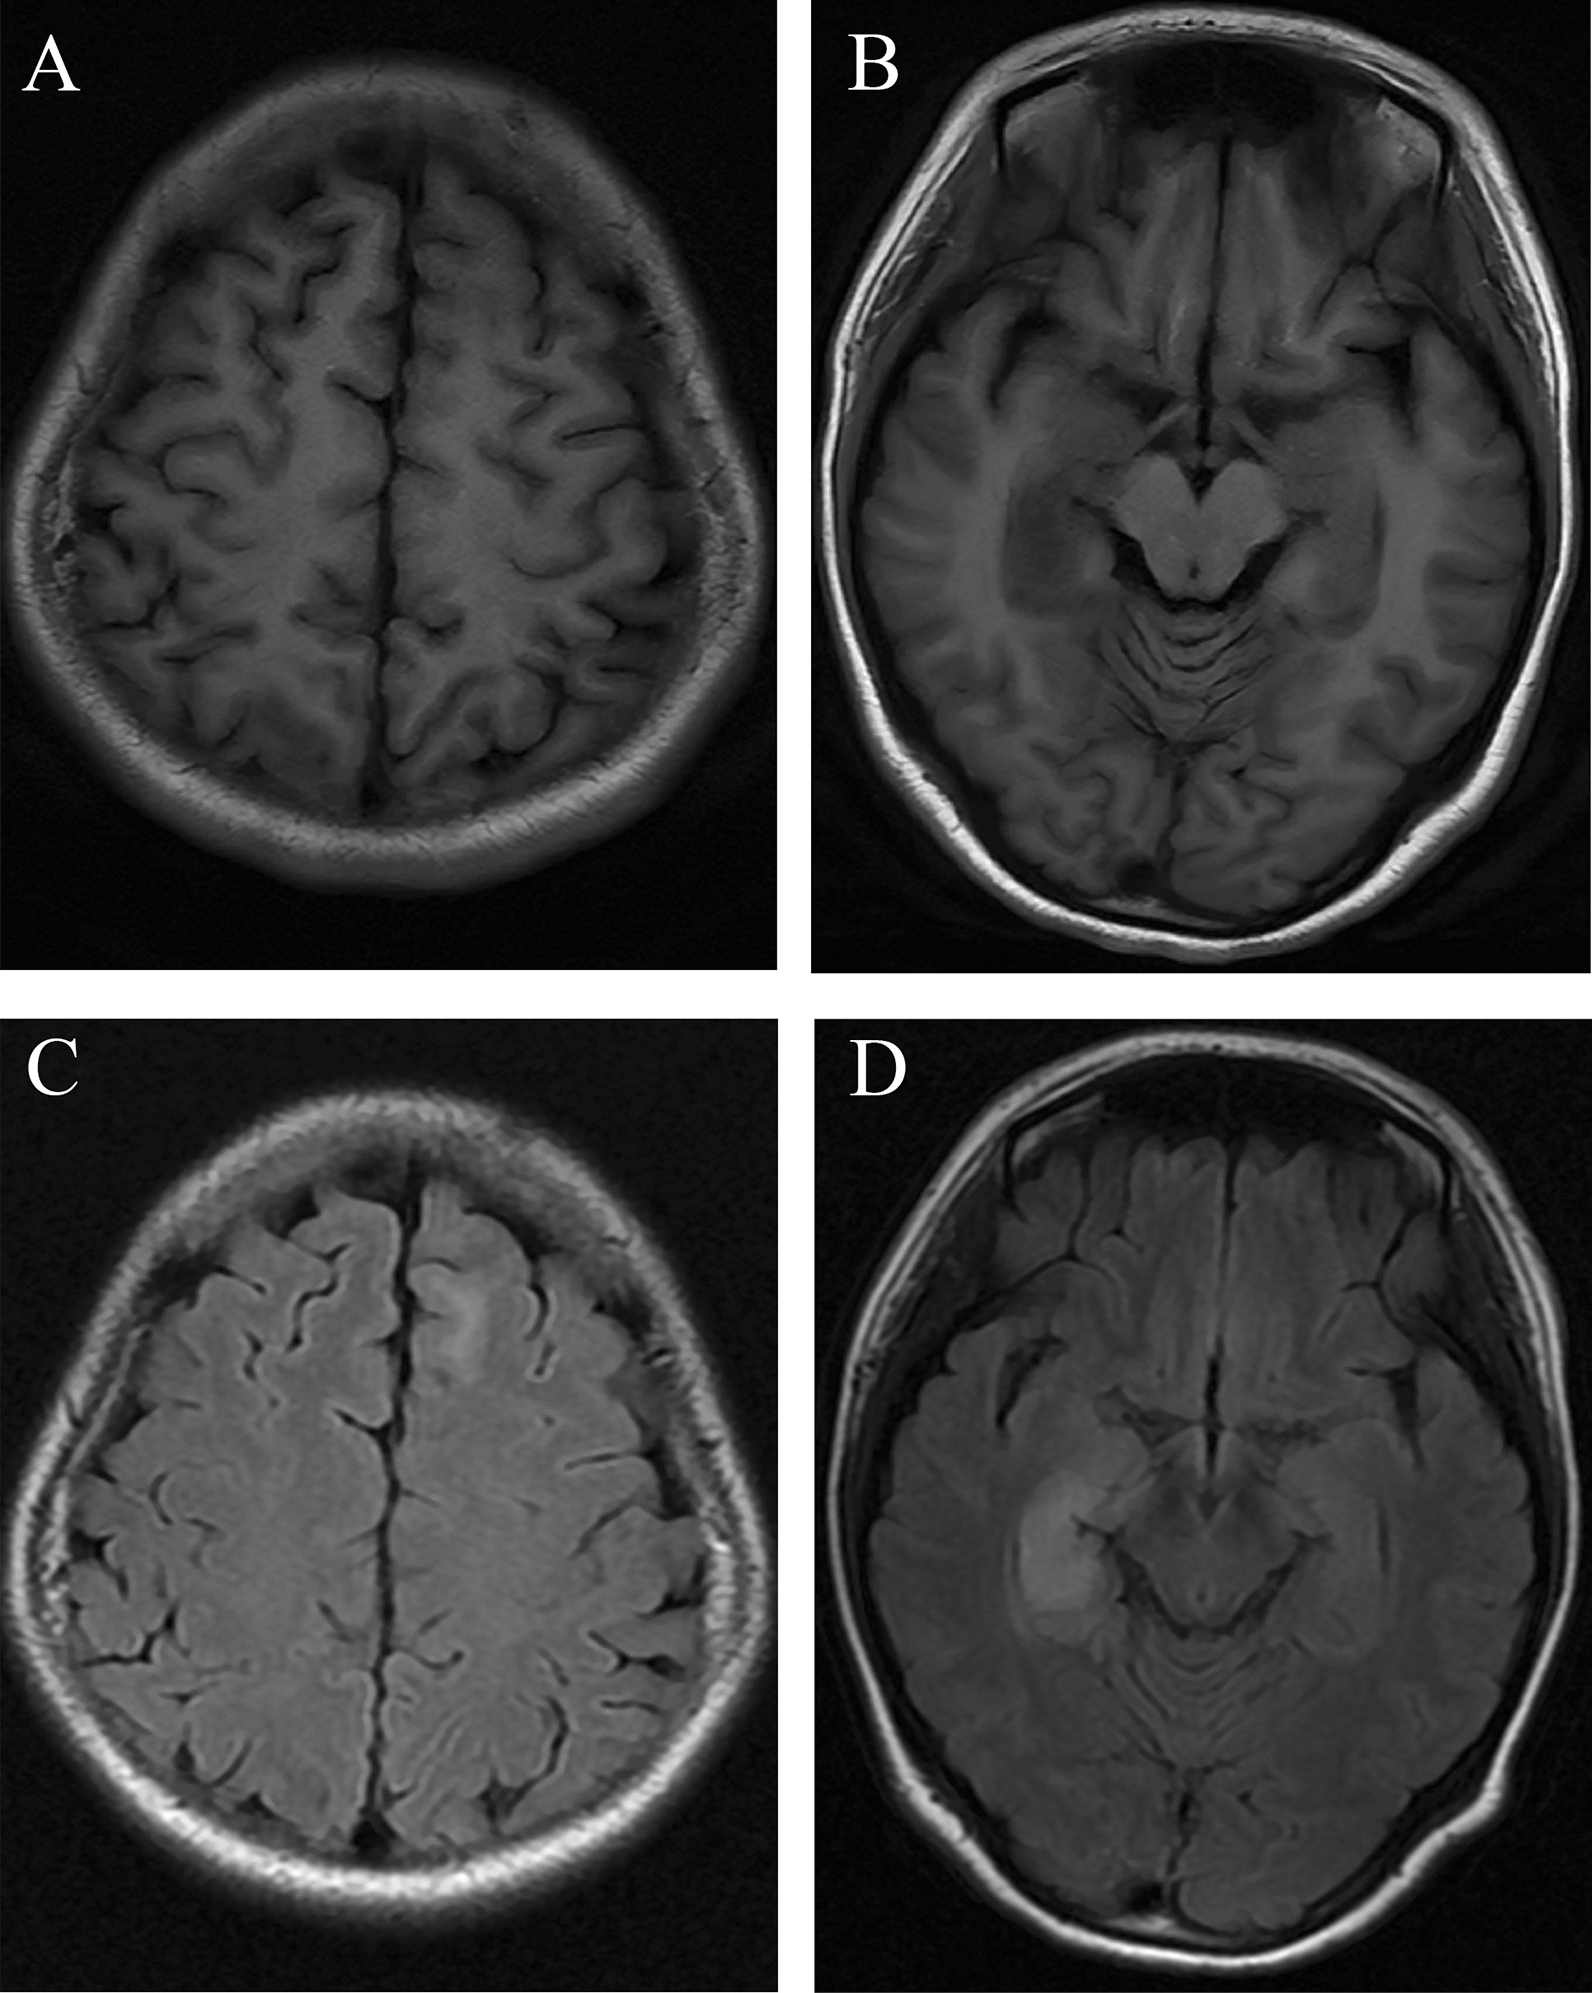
Brain magnetic resonance imaging findings of a young female patient with anti-leucine-rich glioma-inactivated 1 encephalitis. Axial T1-weighted imaging A, B showed hypointensities in left frontal lobe and right medial temporal lobe, with corresponding hyperintensities on axial fluid attenuated inversion recovery imaging C, D
